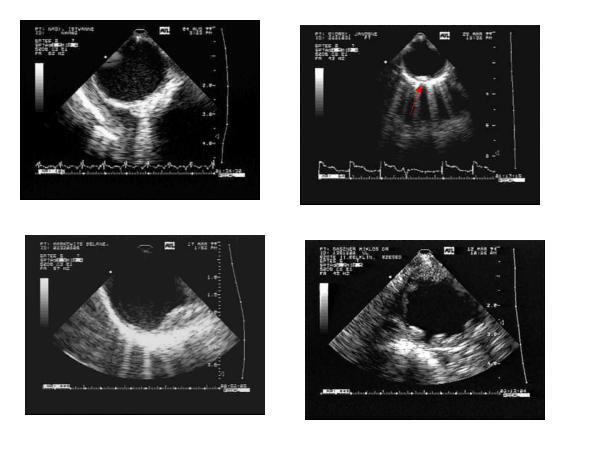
Grading of the transesophageally detected aortic lesions in the descending aorta. Grade 1: intimal thickening (left upper panel); Grade 2: small plaque indicated by arrow right upper panel; Grade 3: lower panels. On the lower panels: left a huge, multiple plaque, right: an ulcerated plaque with mobile part.
